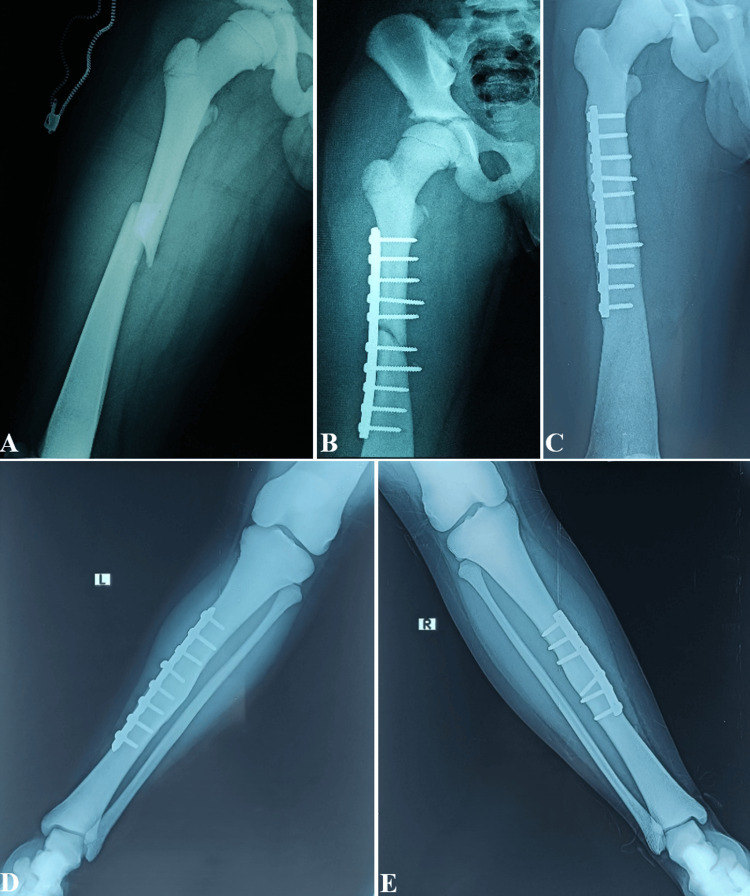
Lower-limb radiographs showing a right femoral fracture sustained at the age of 15 years (A), treated with plate fixation, with immediate postoperative imaging (B) and the six-year follow-up imaging (C). Follow-up radiographs obtained two years after surgery show left tibial (D) and right tibial (E) fractures sustained at the age of 18 years.
